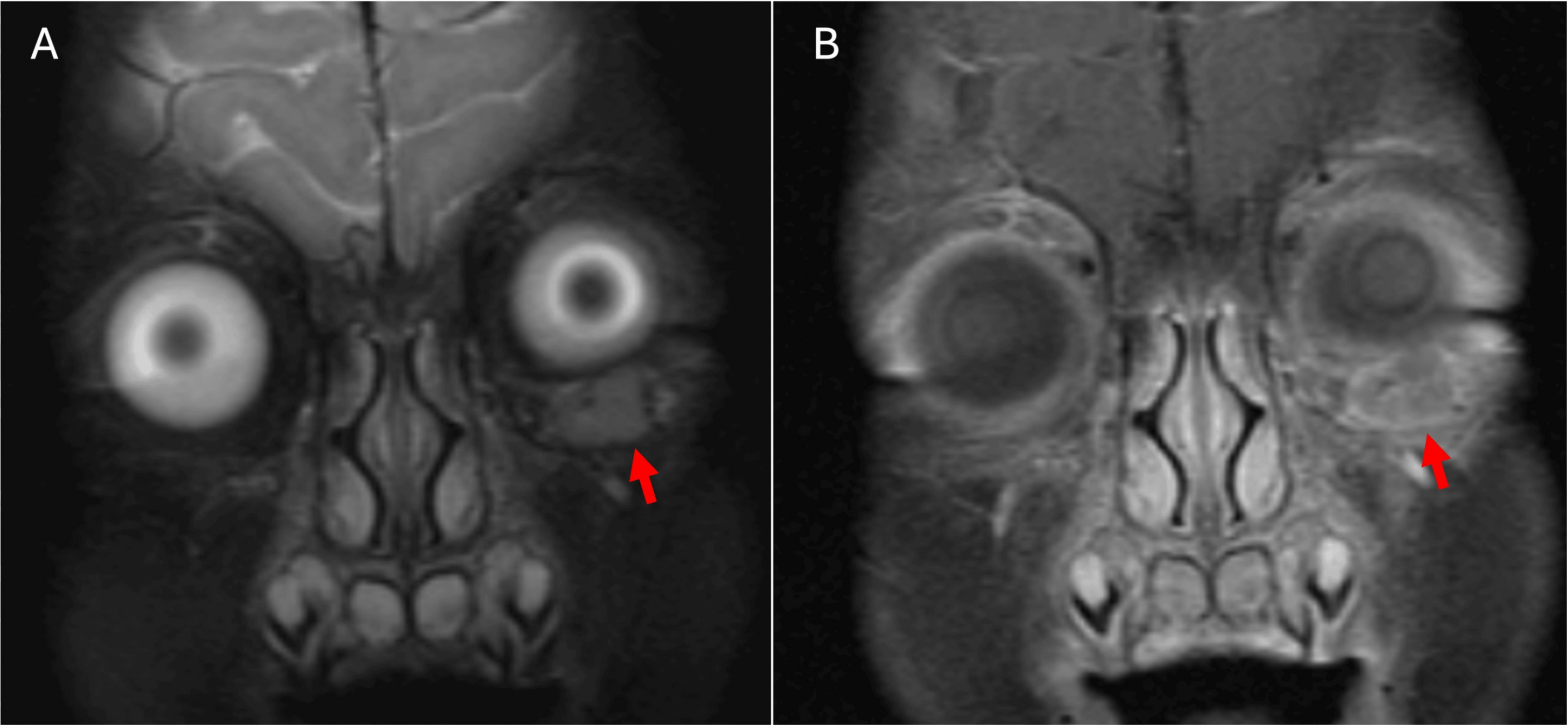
Coronal T2-weighted (A) and T1-weighted post-contrast (B) images performed seven weeks after resection demonstrate a new T2 isointense to mildly hyperintense enhancing lesion in the left inferior eyelid (arrow), which was confirmed to represent a site of recurrence upon re-resection.
